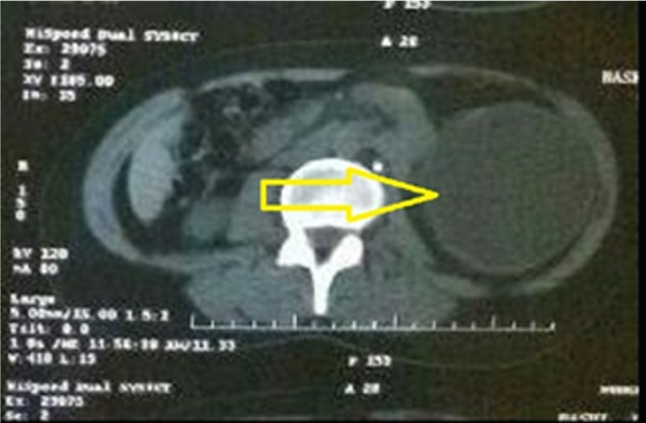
CT scan for kidneys. Right kidney with a normal dimension of 23×121 mm and without stone or obstruction. Left kidney with a dimension of 18×165 mm and the existence of a typical cyst with a dimension of 93×120 mm.
